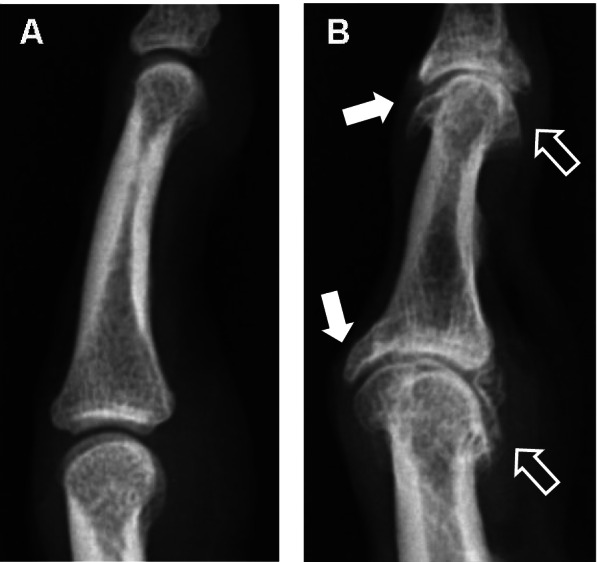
Example of lateral radiographic views of distal interphalangeal (DIP) and proximal interphalangeal (PIP) joints. A Normal DIP and PIP joints without dorso-ventral osteophytes (dvOPs). B DIP and PIP joints displaying dvOPs. The white arrows indicate dorsal osteophytes (OP), the outlined arrows mark ventral OPs
